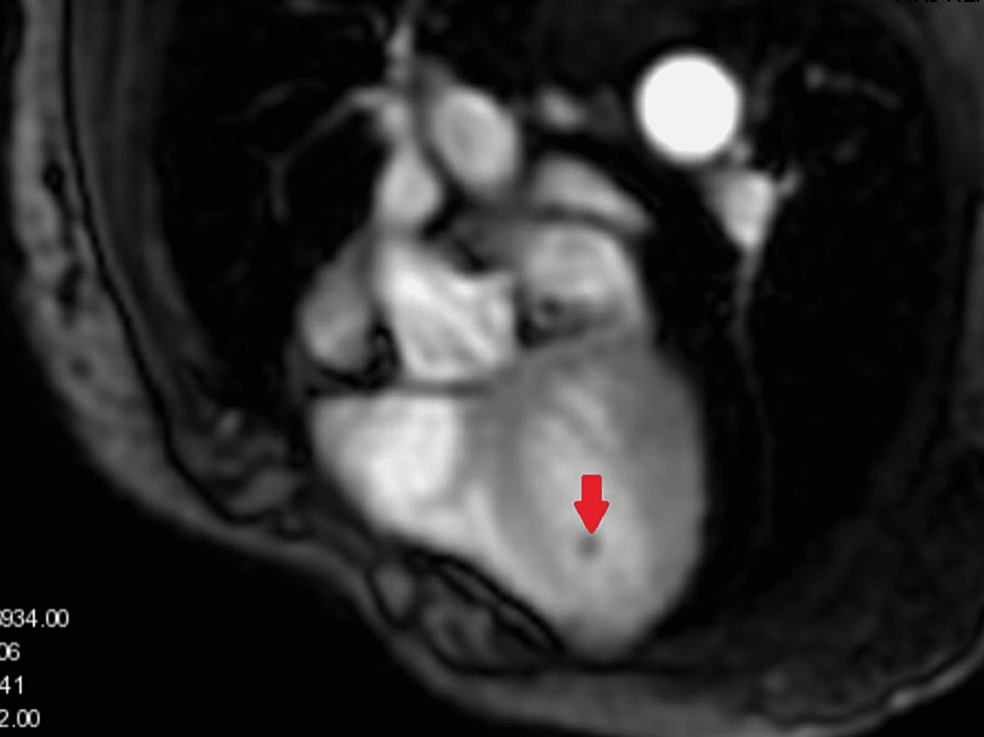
Cardiac MRI demonstrating a filling defect in the left ventricle consistent with a thrombus (red arrow)
